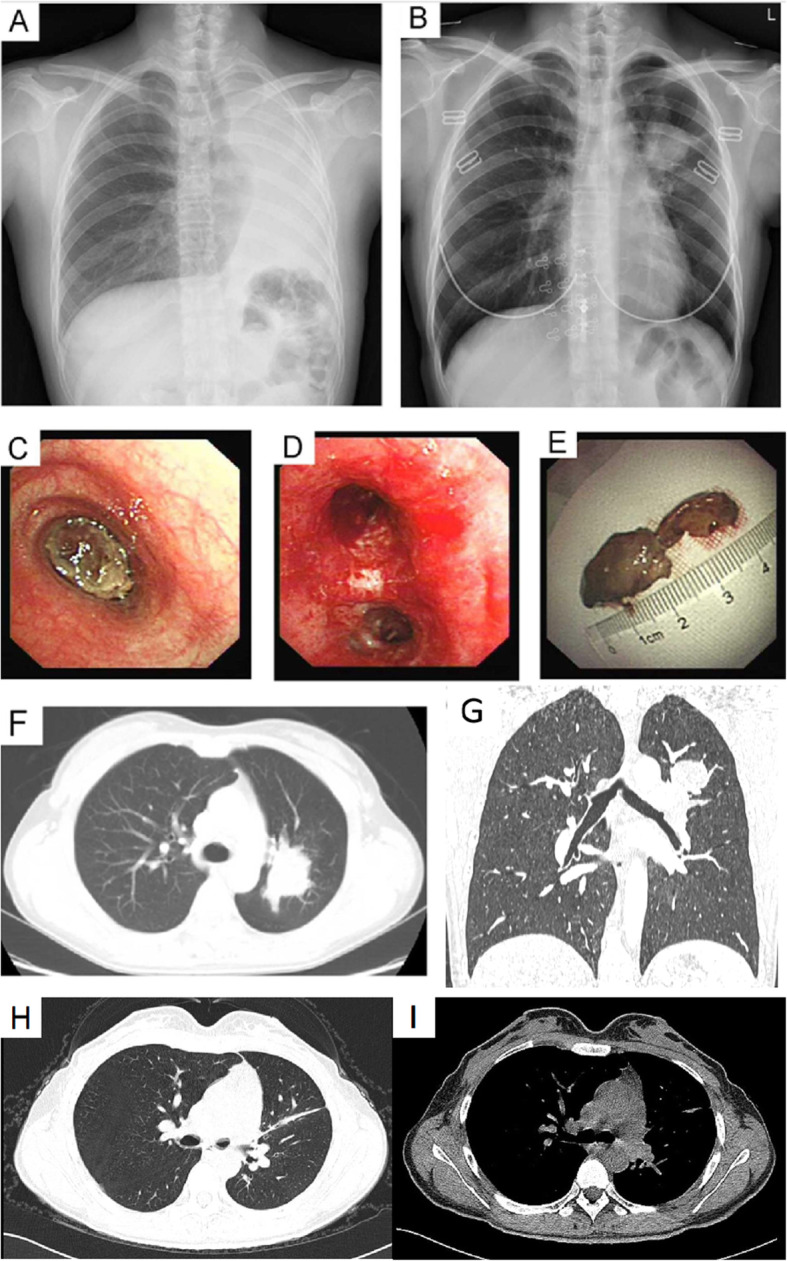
Pulmonary mass. a: Left lung atelectasis was established by chest X-ray test. b: Chest X-ray showed a re-expansion of the left lung and a mass in the left upper lobe after interventional therapy. c: Bronchoscopy showed a tumor that completely obstructed the left main bronchus. d: The interventional therapy fully re-opened the obstructed left main bronchus and the lower subsegment of the left lower lobe. e: The lesion was partially resected by loop electrocautery via flexible bronchoscopy. f: Chest CT scan showed a tumor on the left upper lobe. g: Coronal CT after loop endoscopically resection on the left upper lobe. h: Chest CT scan showed the residual scar tissue after surgery a week later. i: CT demonstrated no relapse after a 6-year follow-up in 2019.12
